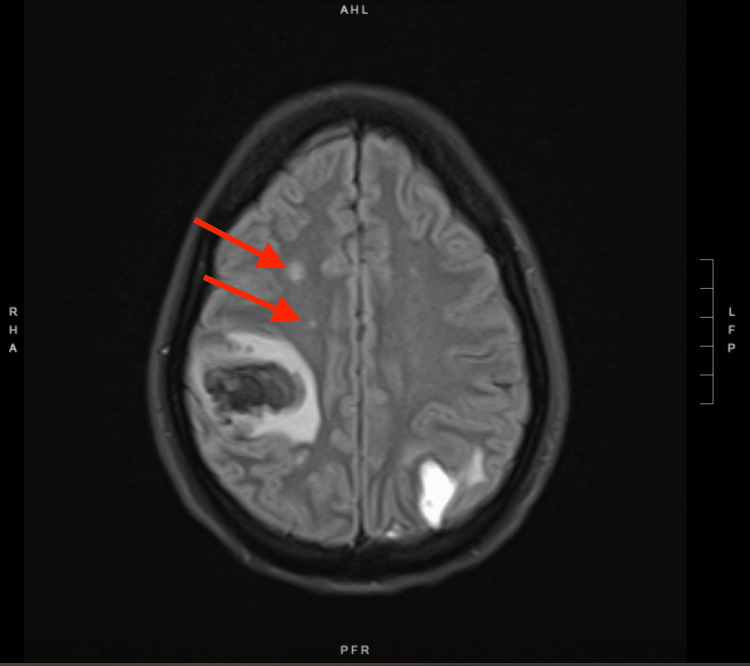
MRI brain Red arrows depict foci of punctate enhancement. Areas of corresponding FLAIR signal abnormality in the bilateral cerebral hemispheres are also visible MRI: magnetic resonance imaging; FLAIR: fluid-attenuated inversion recovery
